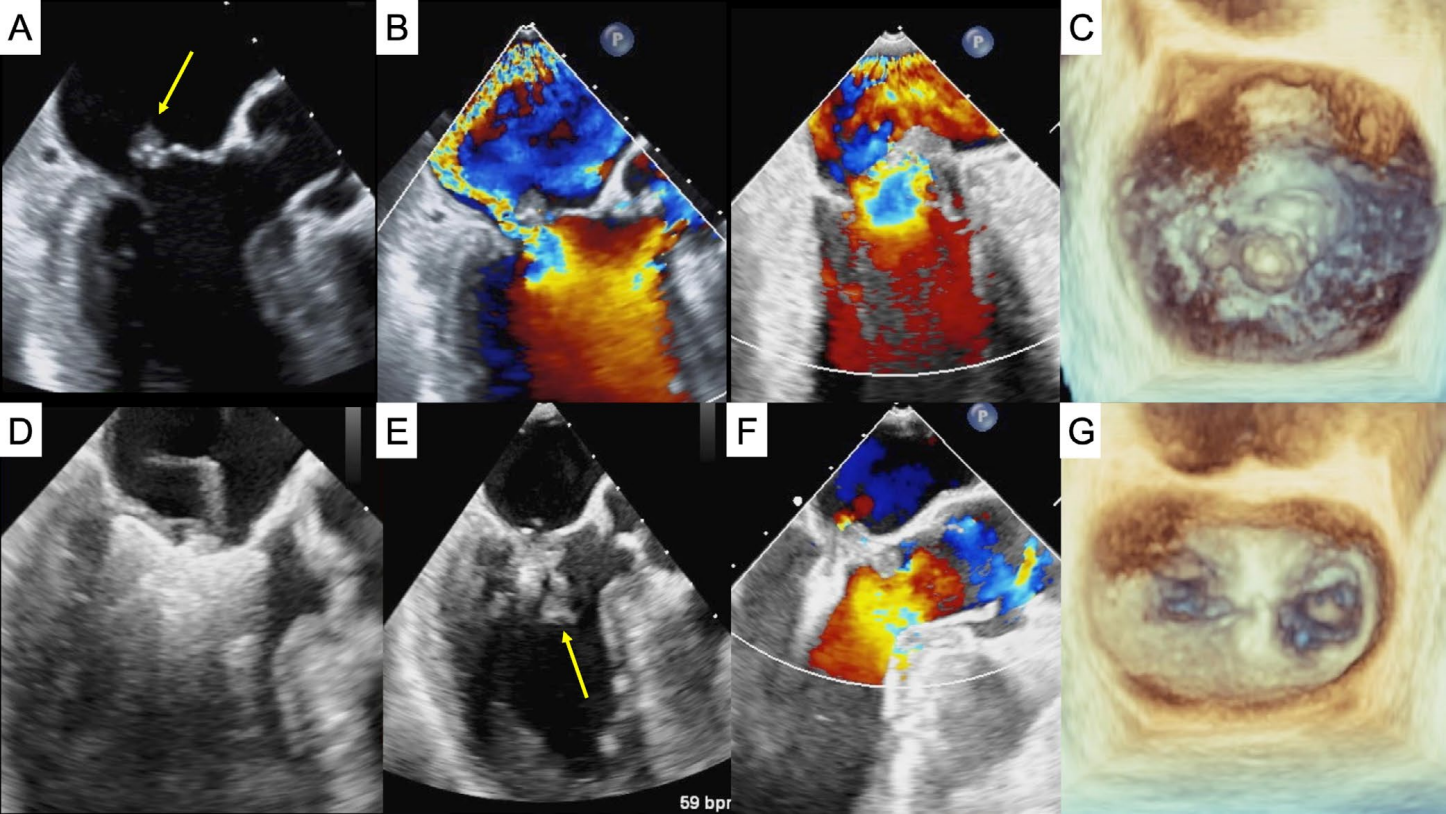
Transcatheter edge‐to‐edge mitral valve repair for papillary muscle rupture. (A) Rupture of the anterior papillary muscle (arrow). (B, C) Preprocedural TEE images, including left ventricular outflow tract views, bicommissural views, and three‐dimensional reconstructions. (D) Both mitral leaflets are securely grasped and deeply inserted into the clip arms. (E) The ruptured papillary muscle (arrow) is visualized within the left ventricle. (F) Postprocedural TEE shows trivial residual mitral regurgitation. (G) Three‐dimensional TEE image demonstrating a single clip creating a tissue bridge. TEE, transesophageal echocardiography.
